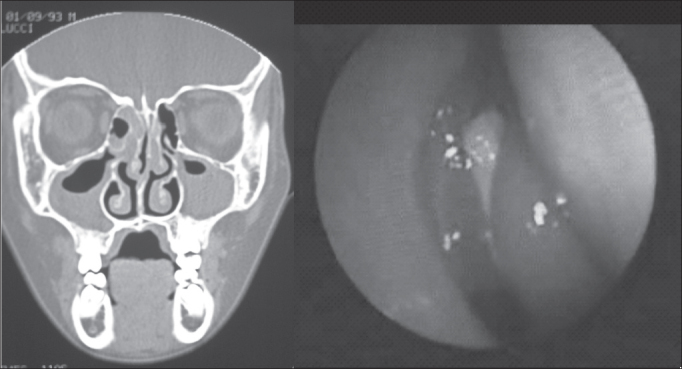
Example (case 12) of the agreement between results of assessments. CT scan and nasofibroscopy with positive diagnosis of chronic rhinosinusitis.
